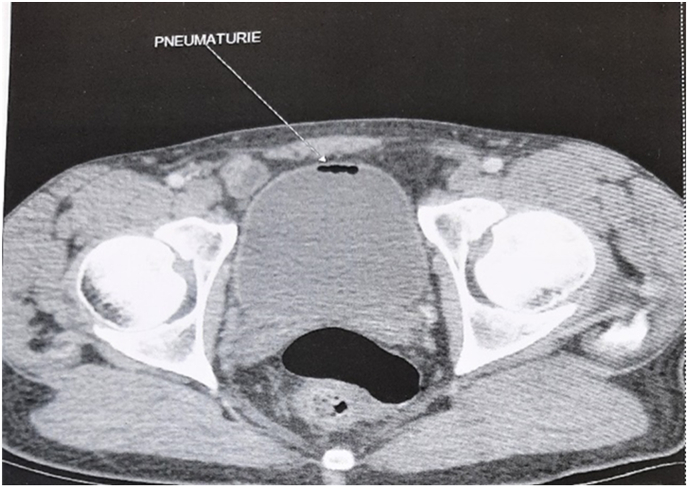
Abdominal CT scan showing bladder pneumaturia.
